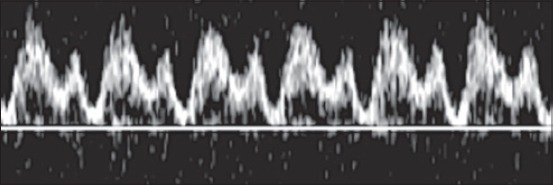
Pulsed-wave Doppler image of a diphasic waveform of a ductus venosus.
